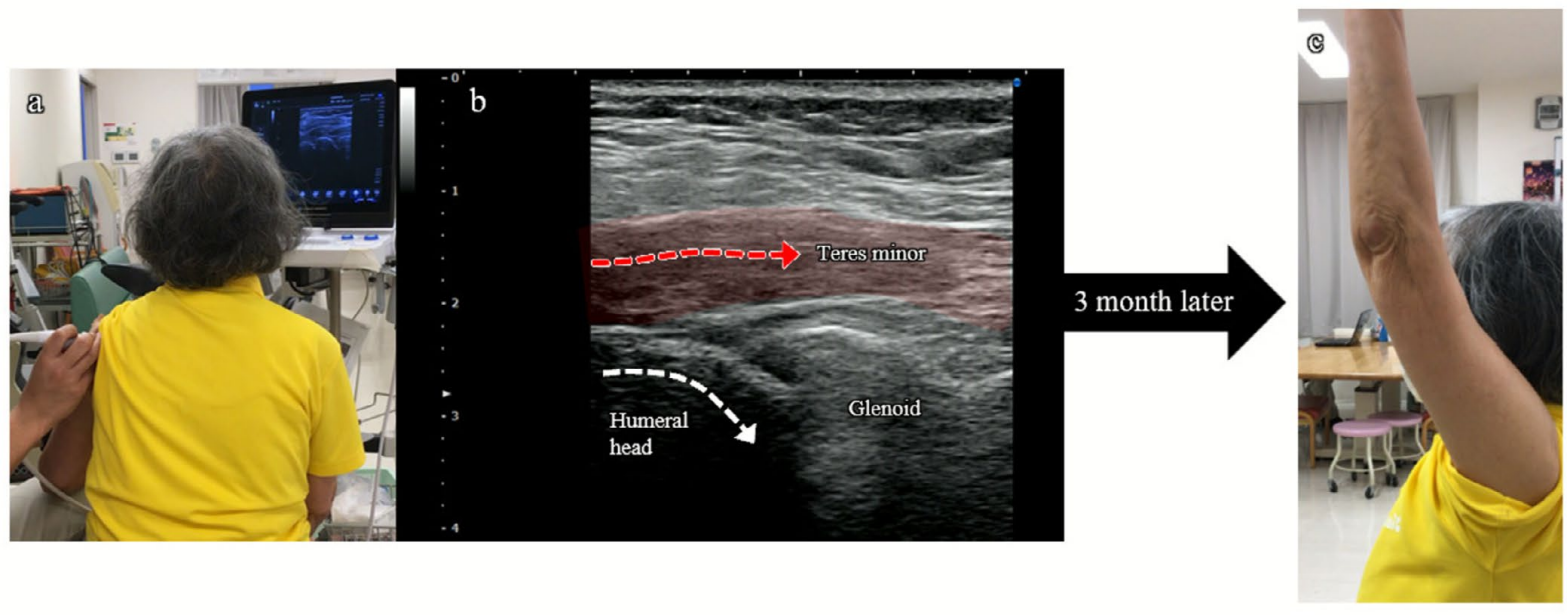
Ultrasound‐guided exercise therapy (long‐axis view to the teres minor muscle) performed under figure‐eight stabilizing shoulder taping. (a) Exercise therapy conducted with real‐time ultrasound feedback while the shoulder was stabilized using figure‐eight stabilizing shoulder taping. (b) Dynamic ultrasound image demonstrating teres minor contraction (red arrow), promoting smooth axial rotation of the humeral head (white arrow) (see also Videos S3 and S4). (c) Clinical outcome at 3 months: active forward elevation improved from 85° to 165°, and pain during motion decreased from NRS 8 to 3.5 without taping.
